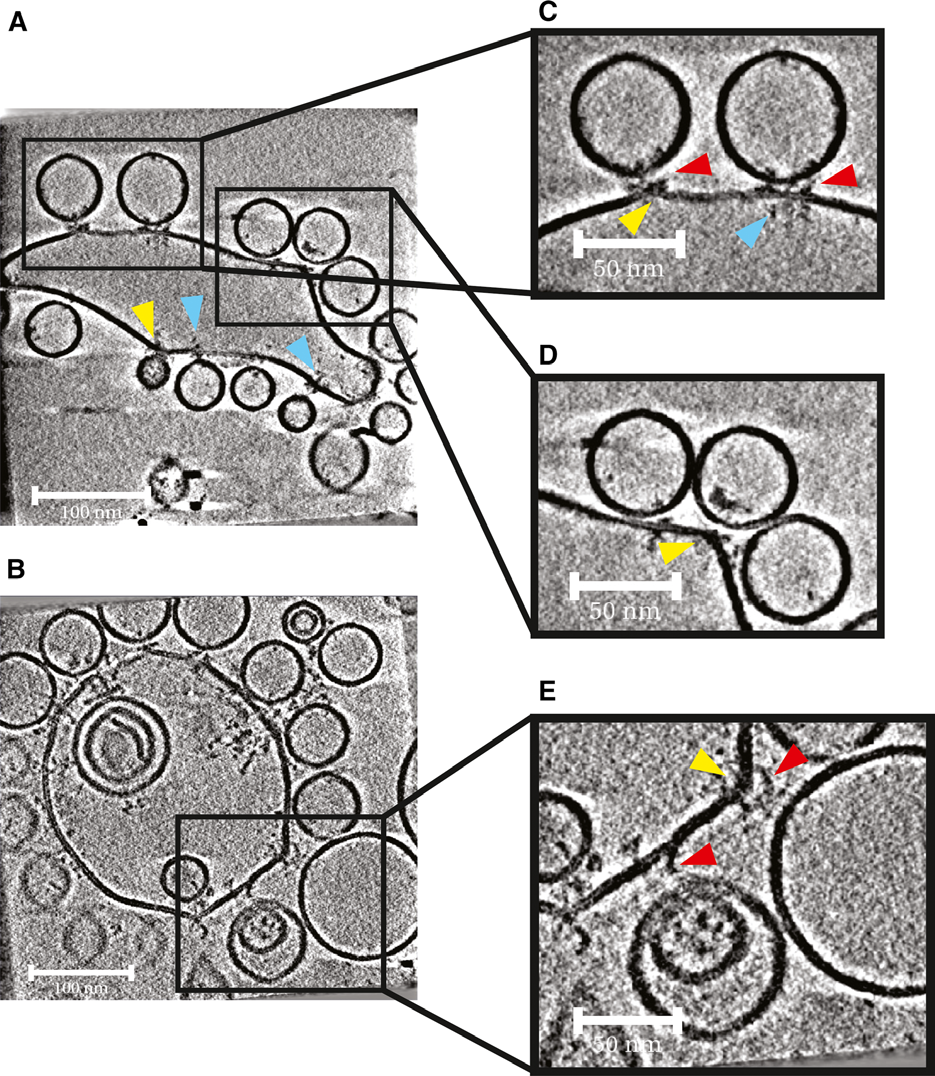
Morphology of vesicle docking sites. Slices from tomograms of sites where SUVs were observed docked to GUVs. Some GUVs appeared more tubulated (A), while other GUVs were more spherical in appearance (B). Protein complexes were observed on the inner leaflets of GUVs clustered around SUV‐GUV docking sites (blue arrowheads). (C) Protein complexes were observed on outer leaflets of GUVs, clustered around sites where SUVs were docked to GUVs (red arrowheads). (D) At sites where SUVs were docked, GUV membrane protrusions were sometimes observed (yellow arrowheads). (E) GUV membrane protrusions were often seen at sites where an SUV was docked at a large distance from the GUV. Scale bars in A and B = 100 nm; scale bars in C, D and E = 50 nm.
